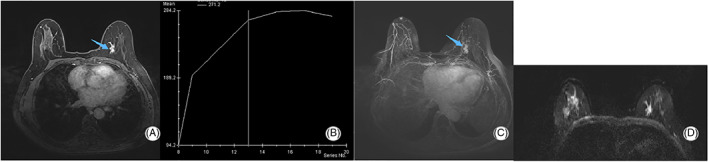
Findings of MRI examination. (A) An area of non‐mass enhancement is detected. (B) A type II enhancement curve is observed. (C) Maximum signal intensity projection image indicates enlarged blood vessels surrounding the lesion in the left breast. (D) Diffusion‐weighted imaging examination reveals mild diffusion limitation
